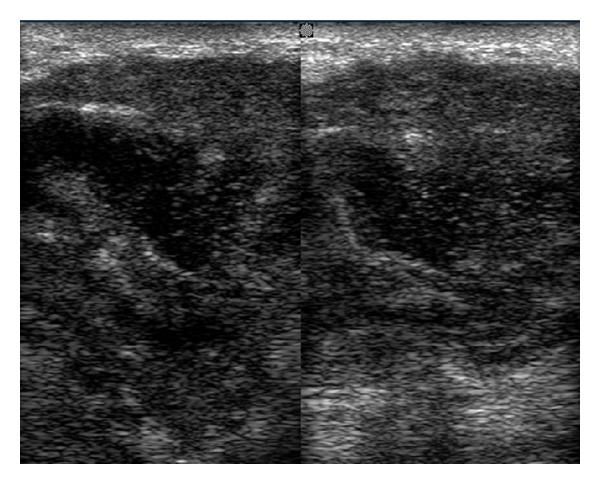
Ultrasonographic findings ill defined hypoecoic lesion.
